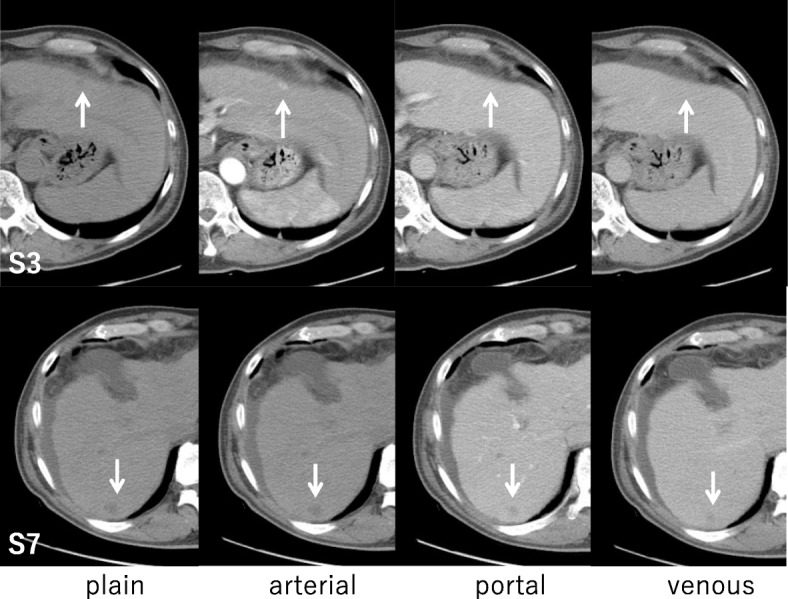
Abdominal computed tomography findings. Pre-contrast computed tomography scans revealed two hypoattenuating hepatic lesions (*arrows*; each ≤1.0 cm in diameter) in segments 3 and 7. The tumor in segment 3 was enhanced in the arterial phase and became isodense to liver parenchyma in the portal and venous phase. The tumor in segment 7 was not enhanced in any phase
